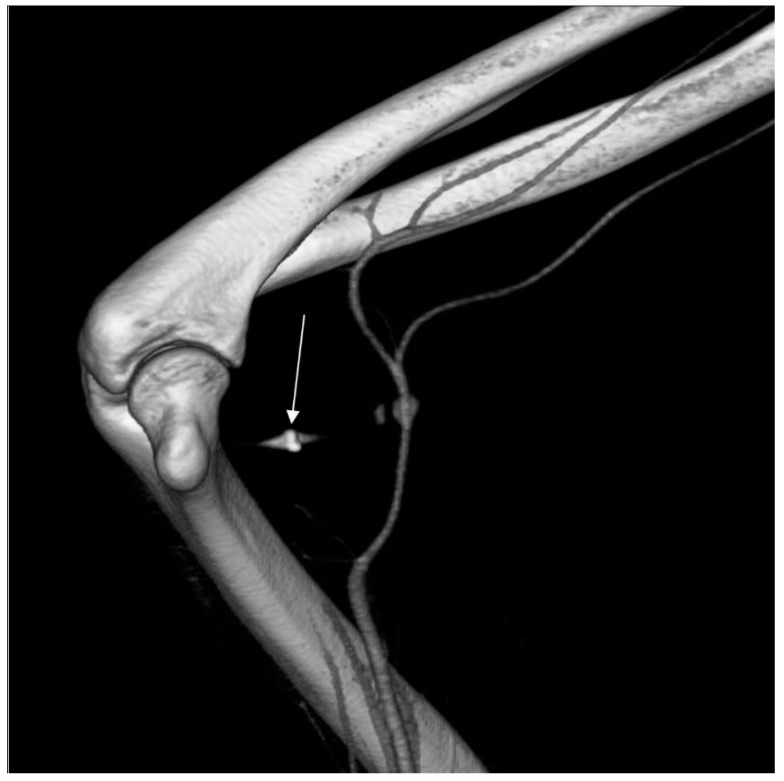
Right upper limb computed tomography angiography. VRT reconstruction. High-density, metallic foreign body within the soft tissue hematoma.
